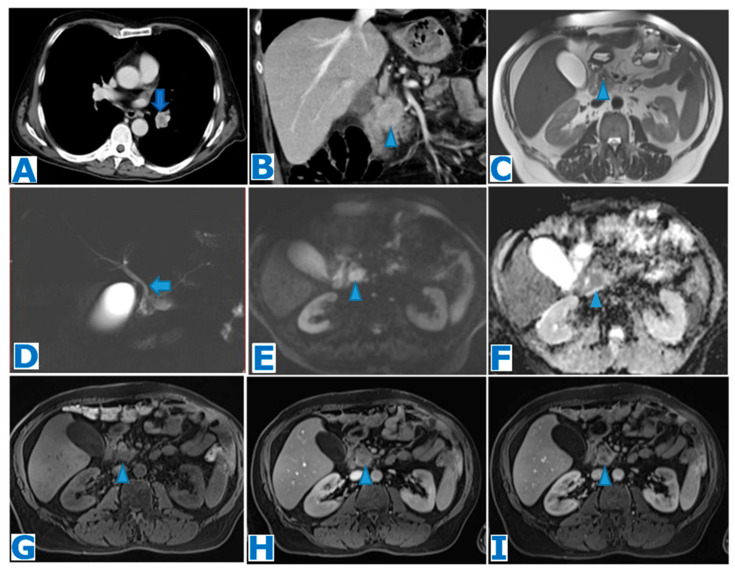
(A): axial CE-CT, (B): coronal MPR CE portal venous phase CT, (C): axial T2WI, (D): MRCP, (E): DWI, (F): ADC, (G): axial NCE FST1WI, (H): axial CE arterial phase FST1WI, (I): axial CE portal venous phase FST1WI. Solitary PM from lung adenocarcinoma in a 56-year-old patient with advanced stage disease and hyperbilirubinemia. Observe the primary tumour within the left hilum (arrow in (A)). The patient also presented peritoneal and bone metastases, not shown. A mass was found within the head of the pancreas (arrowhead in (B)), with no MPD dilatation (arrowhead in (C)). However, the lesion was associated with discreet common bile duct dilatation (arrow in (D)). Observe the diffusion restriction (arrowhead in (E,F)) and the progressive peripheral enhancement following intravenous contrast administration (arrowhead in (G–I)) with central necrosis. Note the resemblance to the primary tumour (arrow in (A)). EUS-guided FNB confirmed a PM from an adenocarcinoma of pulmonary origin.
